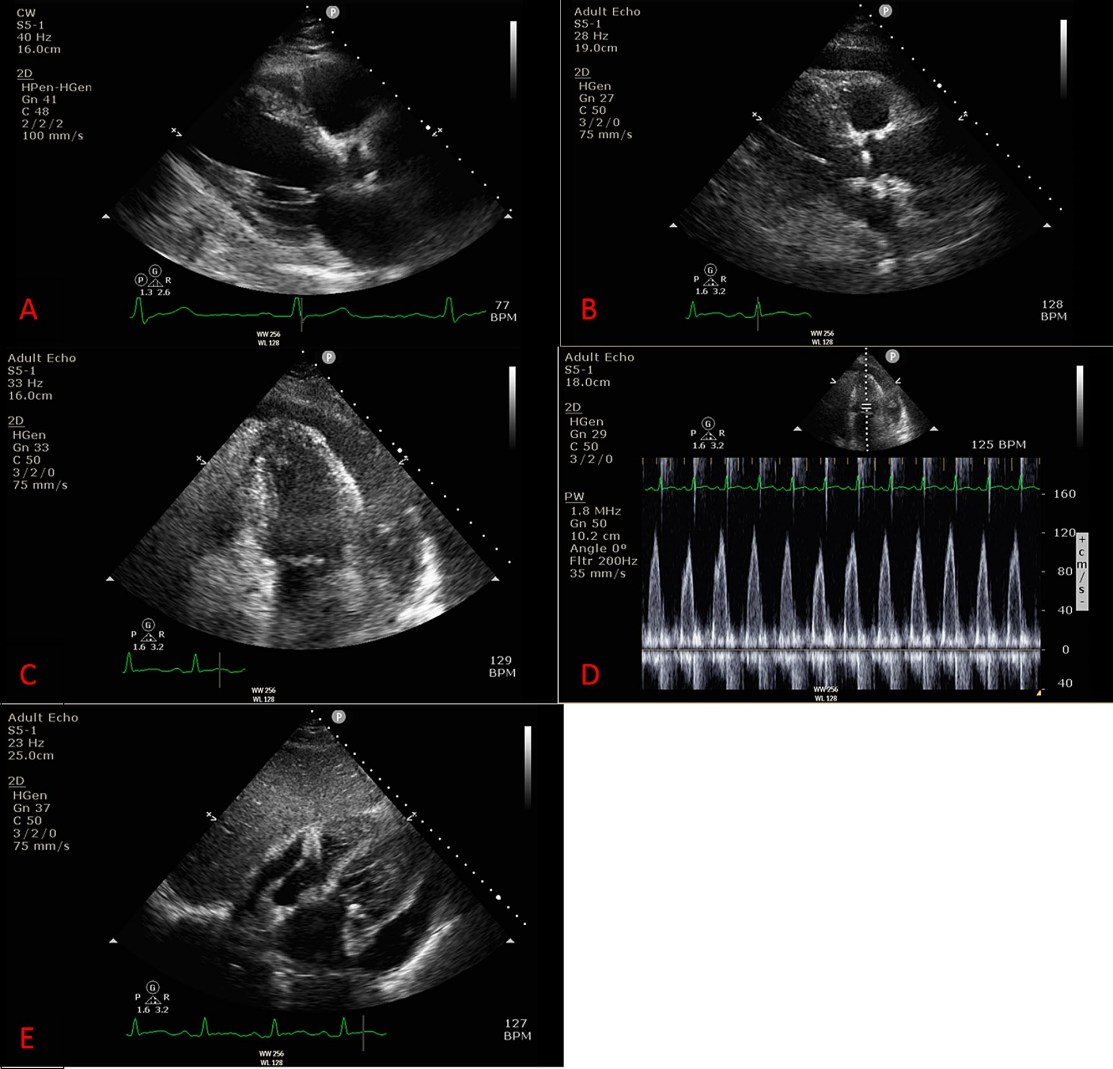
Echocardiographic Images Transthoracic echocardiogram on hospital day #1 showing no significant pericardial effusion on parasternal long axis (A). Repeat echocardiogram on hospital day #3 showing a large pericardial effusion on parasternal long axis (B) and apical (C) views. Mitral inflow variability on pulsed wave Doppler was present (D) along with right atrial wall collapse (E), concerning for a hemodynamically significant effusion
